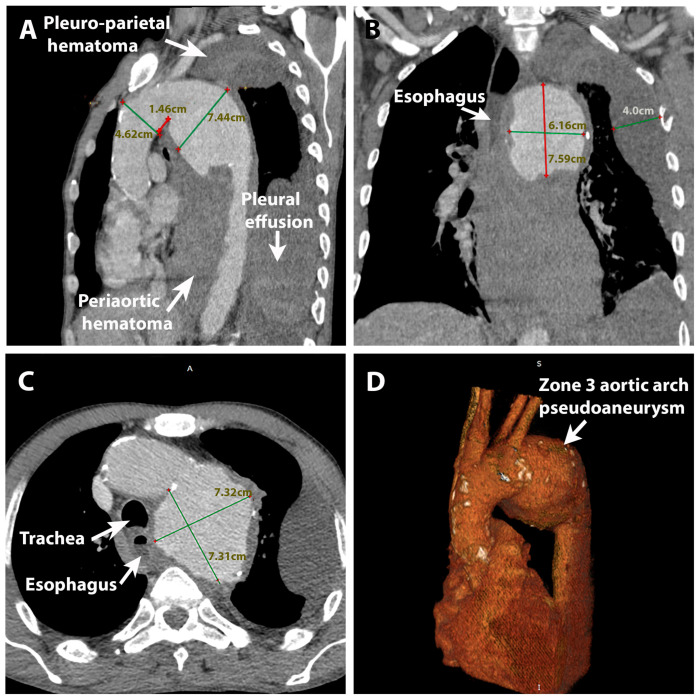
Preoperative angio-CT: (A) distal aortic arch pseudoaneurysm with periaortic hematoma, pleural effusion, and pleuro-parietal hematoma (sagittal plane); (B) (coronal plane); (C) (transverse plane); (D) angioCT reconstruction of the aorta with patchy atherosclerotic calcifications and distal arch pseudoaneurysm.
